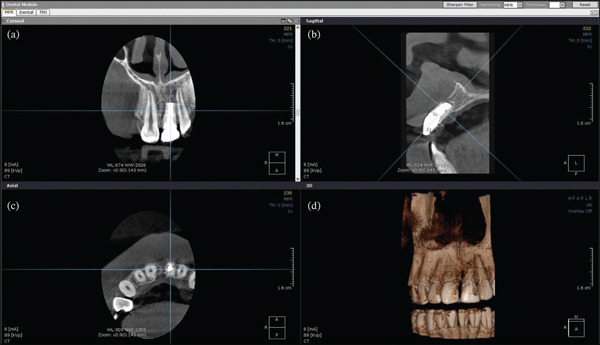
Follow‐up examination after surgical intervention by cone beam computed tomography. (a) Coronal, (b) sagittal, (c) axial, and (d) 3D reconstructions showing bone neoformation indicating repair in the periapical region.
